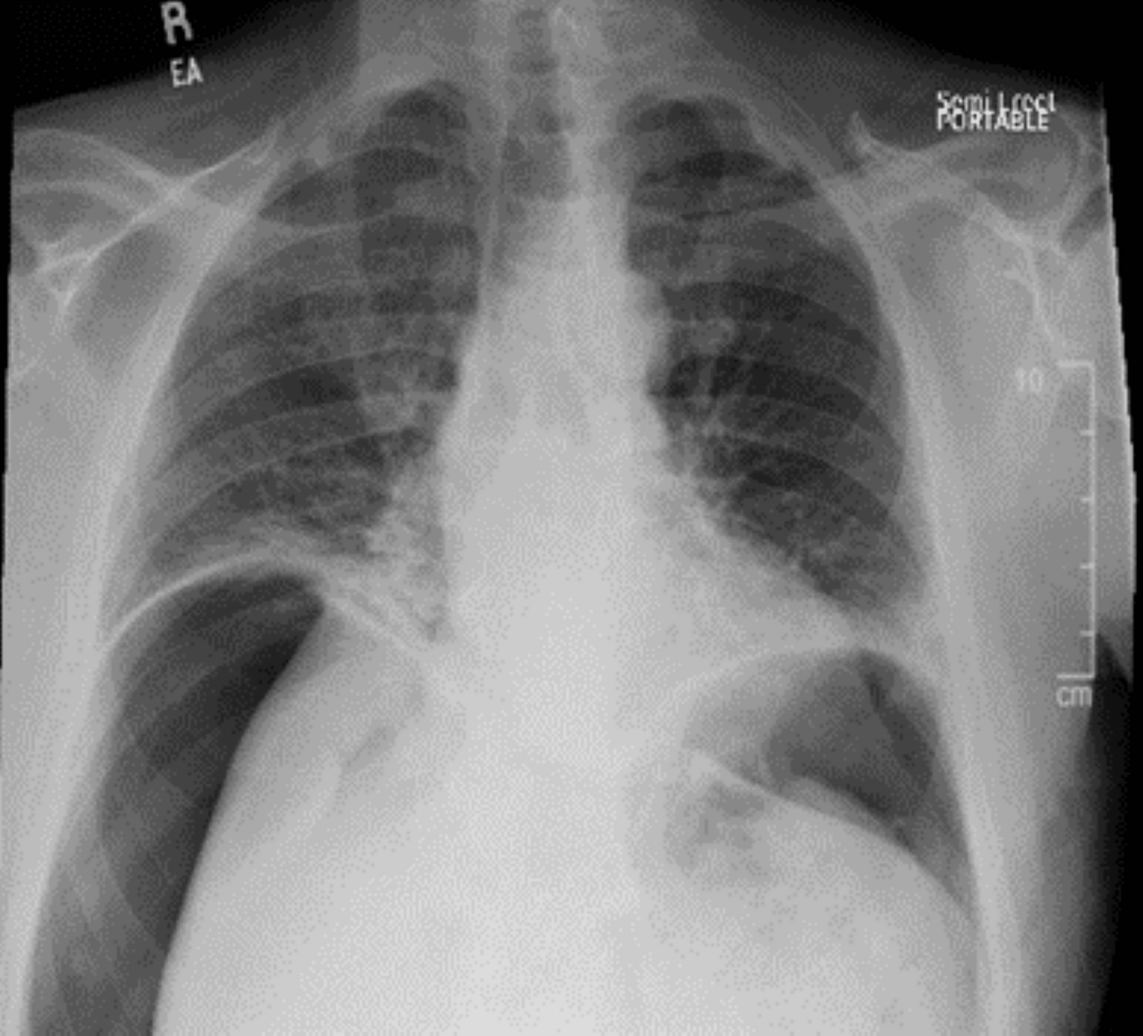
Chest x-ray showing pneumoperitoneum with bilateral subdiaphragmatic air
